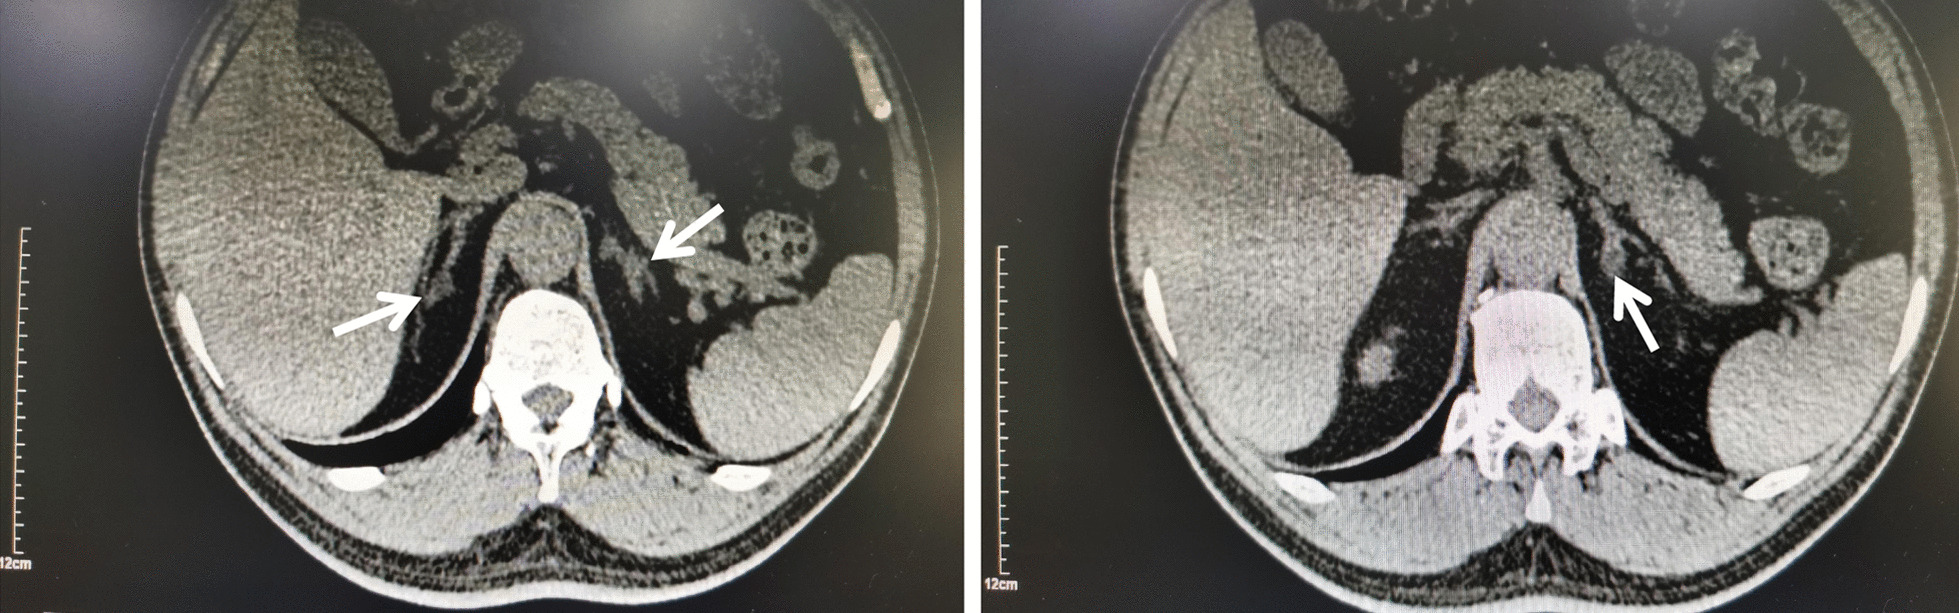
Adrenal computed tomography (CT) scan. The arrows indicate the adenoma
